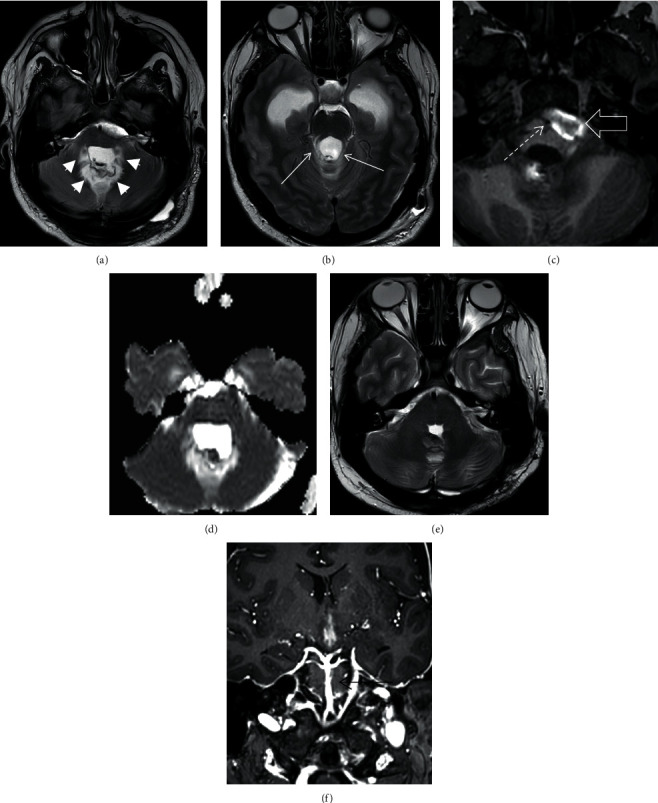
Axial T2 weighted images (a, b) from the MRI performed on day 14 demonstrate bilateral hyperintensity in the regions of the dentate nuclei, middle cerebellar peduncles (white arrow heads), and the superior cerebellar peduncles (white arrows). The T1 weighted image (c) demonstrates the narrow basilar artery (white dashed arrow) and the adjacent haematoma (white open arrow). The ADC image (d) demonstrates unrestricted diffusion in the region of the T2 abnormalities. MRI scan performed 6 weeks following surgery demonstrates complete resolution of the T2 abnormality (e) and normal calibre of the basilar artery (black arrow) on the post-contrast image (f) as compared to the changes seen on Figure 2(c).
